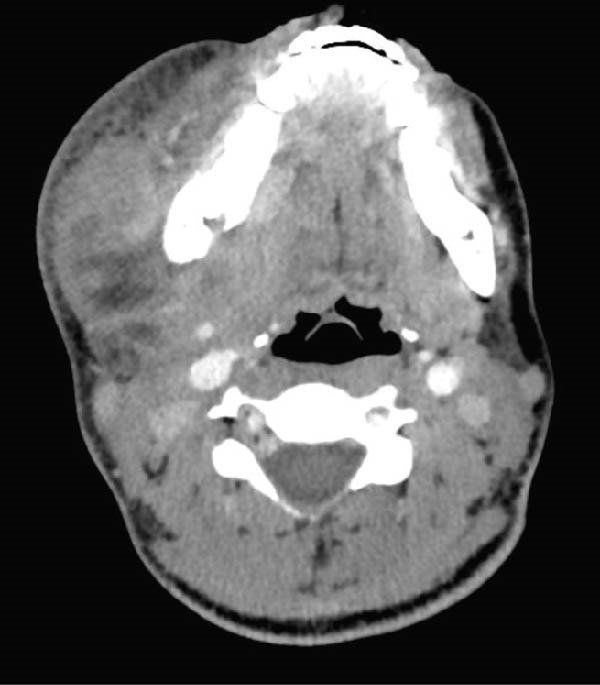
Contrast‐enhanced CT of Case 3 demonstrating multiple collections involving the submental, right pterygomandibular, right submasseteric, and right submandibular spaces.
